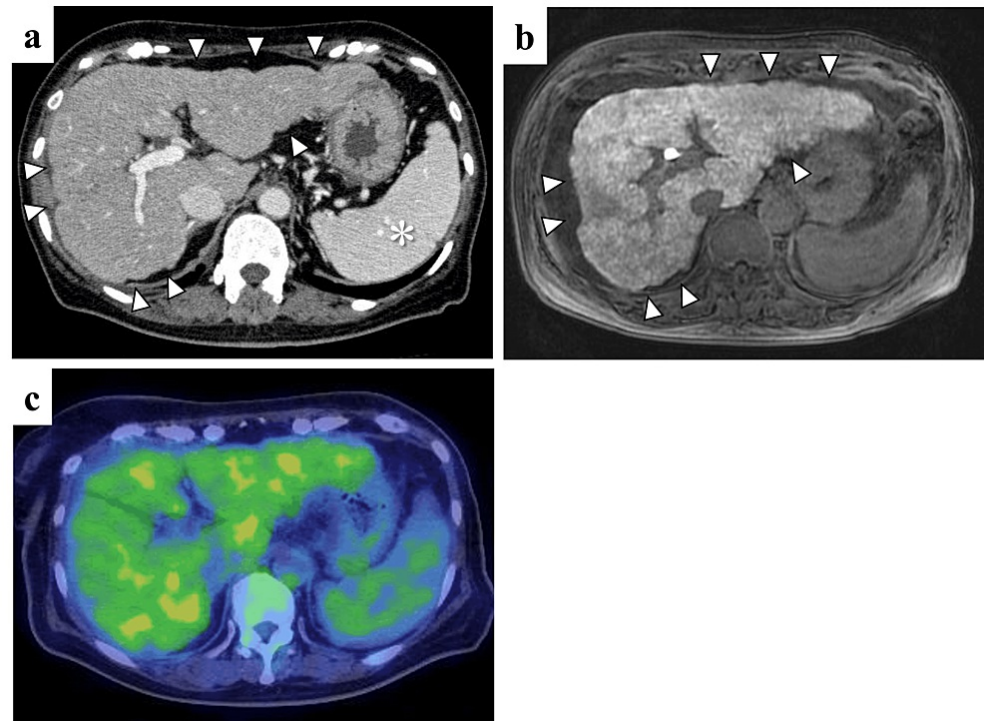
Abdominal images at the time of referral (a, b) Repeated CT and the hepatobiliary phase of gadolinium-ethoxybenzyl-diethylenetriamine-pentaacetic acid-enhanced magnetic resonance imaging showed irregularity of the liver surface (arrowheads), ascites, and splenomegaly (*); however, there were no findings of hepatic metastases. (c) 18F-fluorodeoxyglucose-labeled positron emission tomography-CT scan showed no focal areas of increased uptake to suggest malignancy.
